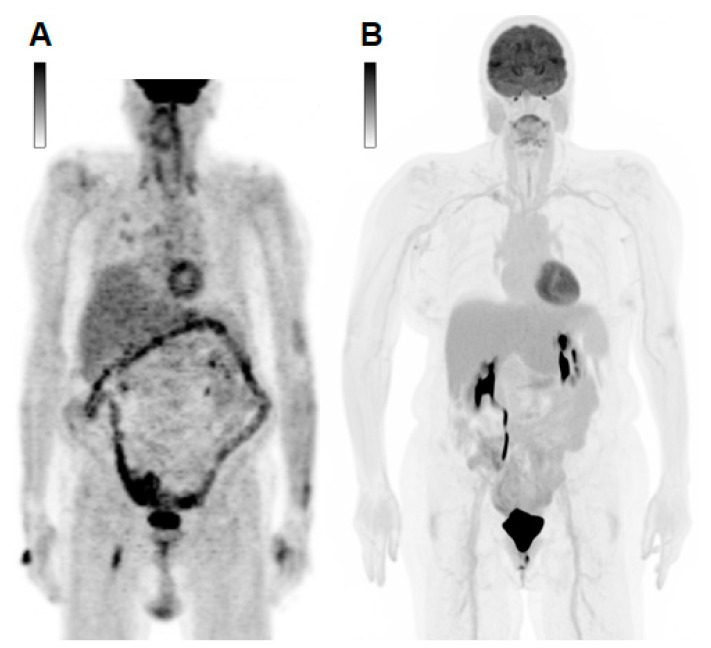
Comparison of imaging from different generations of PET/CT scanners. Maximum intensity projections (MIP) of a conventional analogue scanner with a 60 min acquisition time in 2002 (A) and a state-of-the-art long-axial field-of-view (LAFOV) digital scanner with a 6 min acquisition time in 2021 (B).
